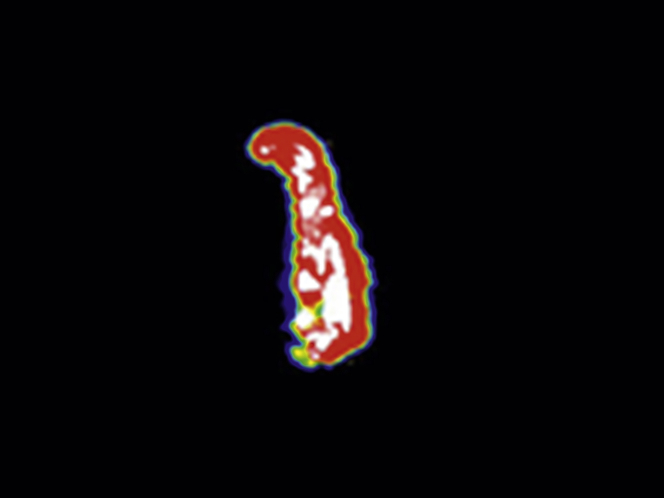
Online Video 1 Micro-positron emission tomography and computed tomography of aortic aneurysm tissue following *ex vivo* incubation with ^18^F-sodium fluoride. Note ^18^F-sodium fluoride uptake (red) by positron emission tomography is distinct from the macrocalcification (white) identified with computed tomography. Some areas of macrocalcification show little or no associated ^18^F-sodium fluoride whereas other areas have avid ^18^F-sodium fluoride uptake in the absence of macrocalcification.
